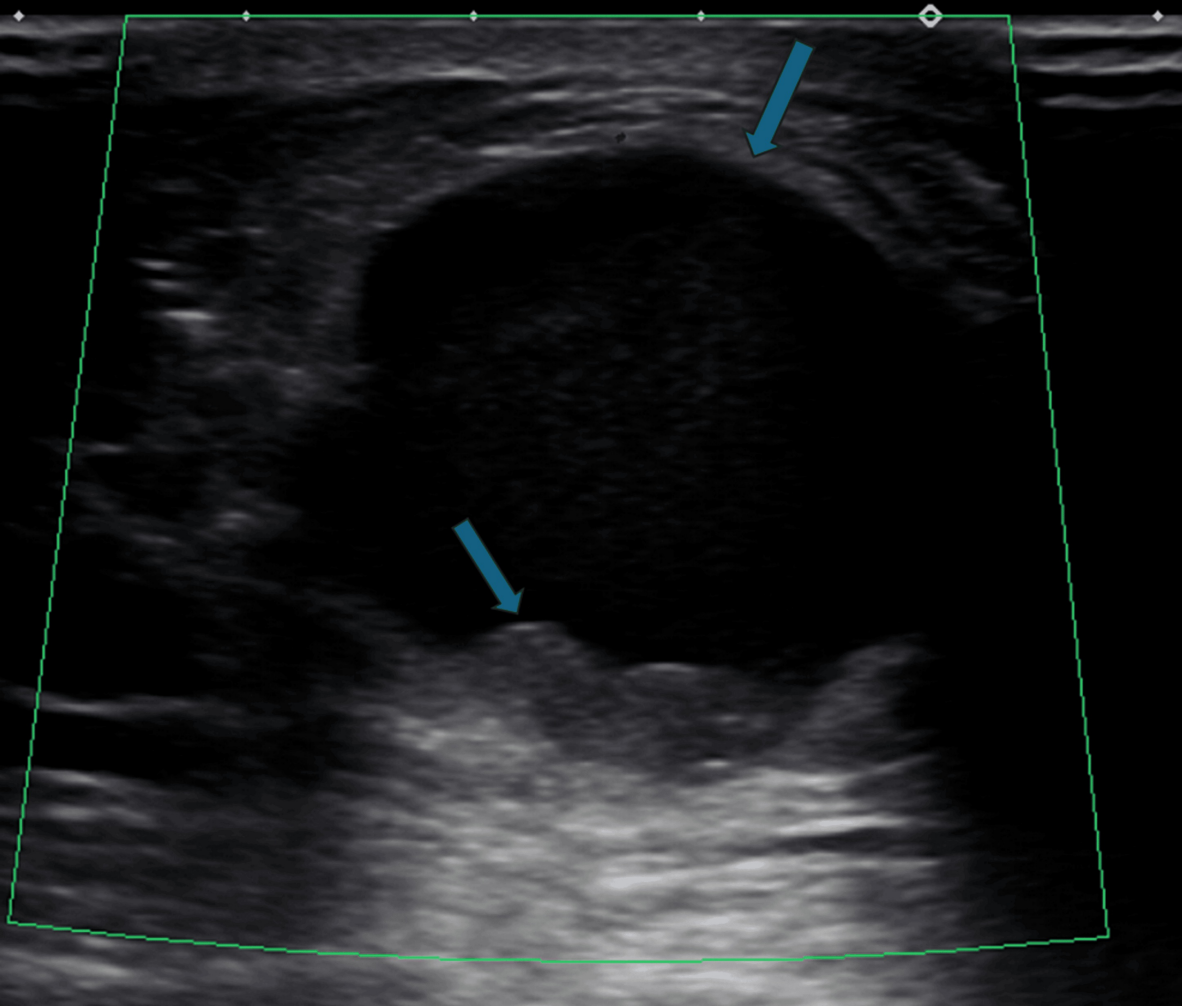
Ultrasound. Grayscale image demonstrating a complex solid and cystic mass in the left subareolar region (blue arrows).
